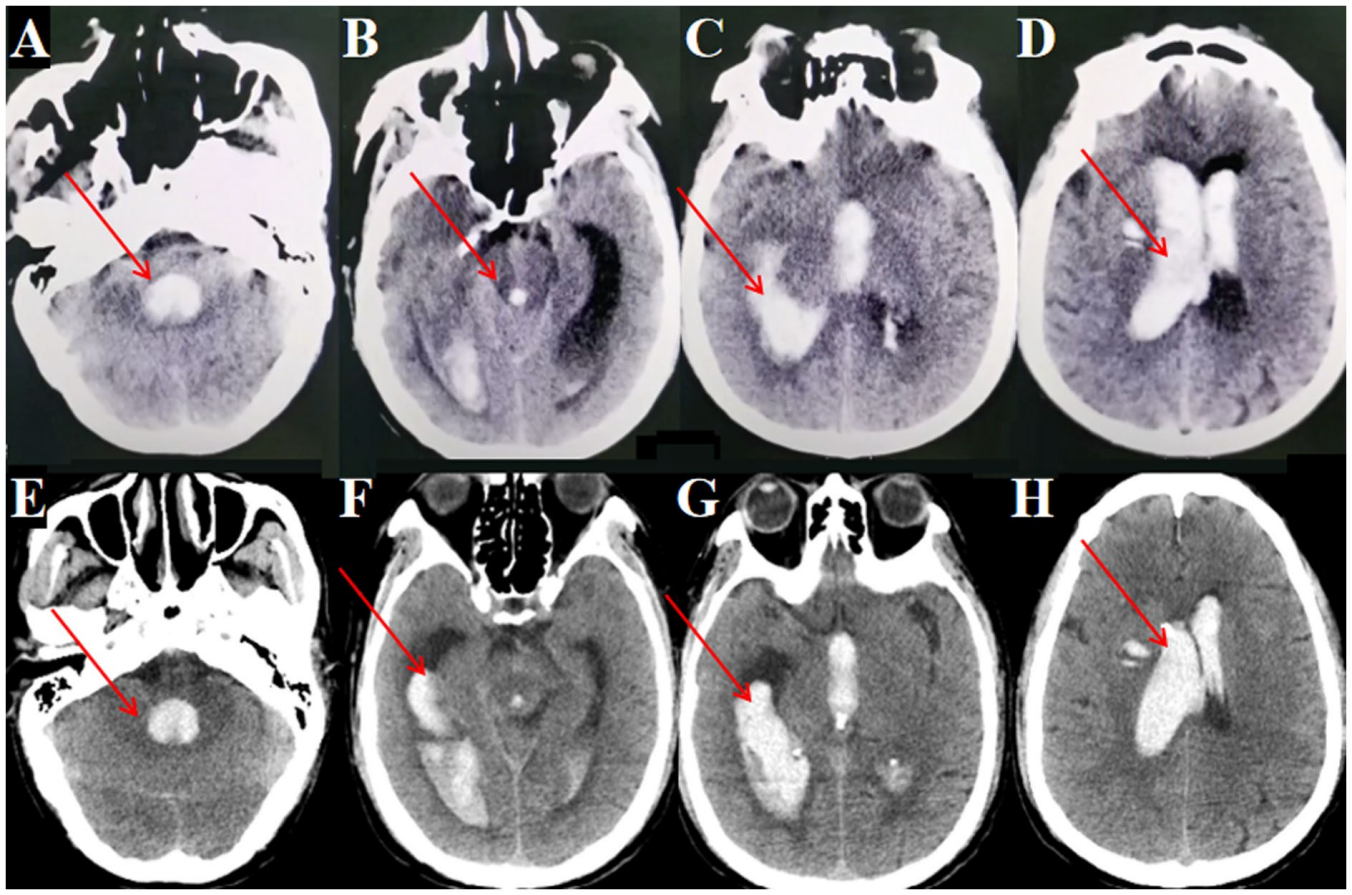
Preoperative and postoperative cranial CT imaging. (A, B) Cranial CT showing fourth ventricular hemorrhage casting (→ arrows indicate blood in the fourth ventricle). (C) Cranial CT showing lateral ventricular hemorrhage casting (→ arrow marks clot in the right lateral ventricle). (D) Cranial CT showing third ventricular hemorrhage casting (→ arrows indicate hematoma in the lateral and third ventricles). (E, F) Postoperative cranial CT after bilateral EVD (performed at local hospital) showing persistent fourth ventricular hemorrhage casting (→ arrows highlight residual clot in the fourth ventricle). (G, H) Postoperative CT after bilateral EVD showing persistent lateral and third ventricular hemorrhage casting (→ arrows mark residual hematoma in the lateral and third ventricles).
